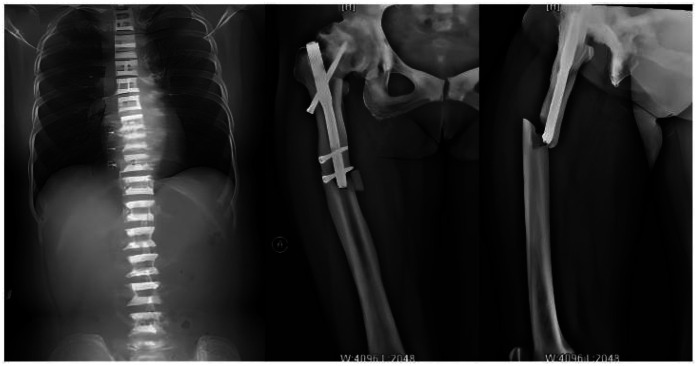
Full-length anteroposterior x-ray film of spine showed that the bone density of upper and lower parts of vertebral body increased, while the density of middle part was lower; preoperative anteroposterior and lateral x-ray images of the right femur show fractures around the internal fixation of the right femur, with signs of hip osteoarthritis and abnormally high bone density.
